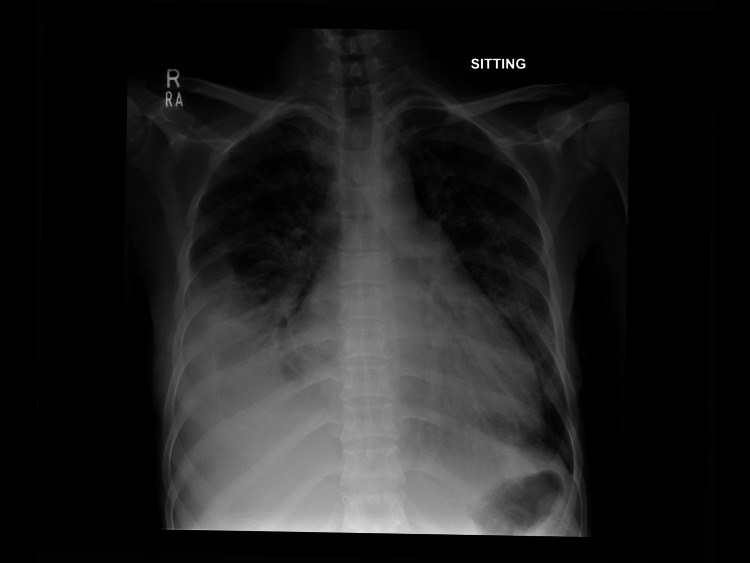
Chest X-ray in AP position showing a magnified cardiac silhouette and moderate right-sided pleural effusion noted with possible underlying atelectasis. There is perihilar congestion with a prominence of upper lobe vessels noted.
